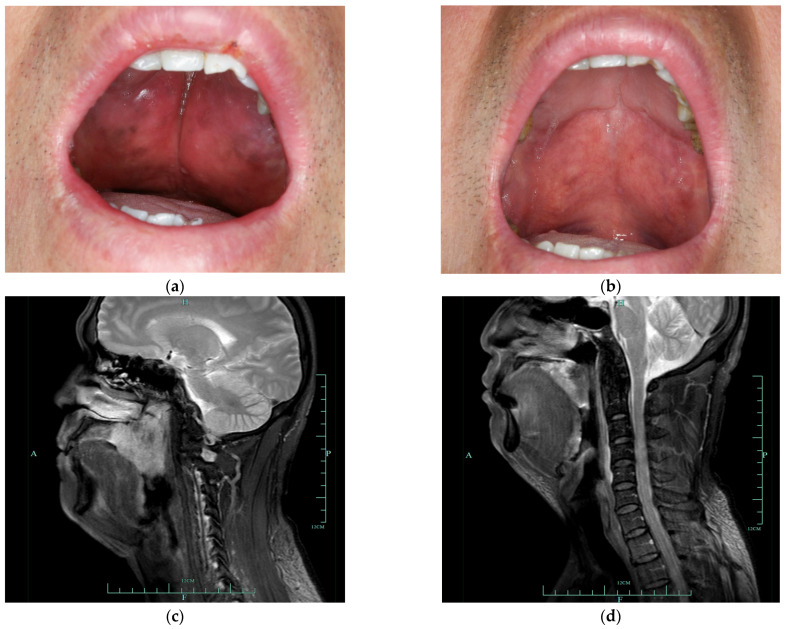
Intraoral photographs and MRI images of the mass before treatment (a,c) and at two months after radiotherapy (b,d).
